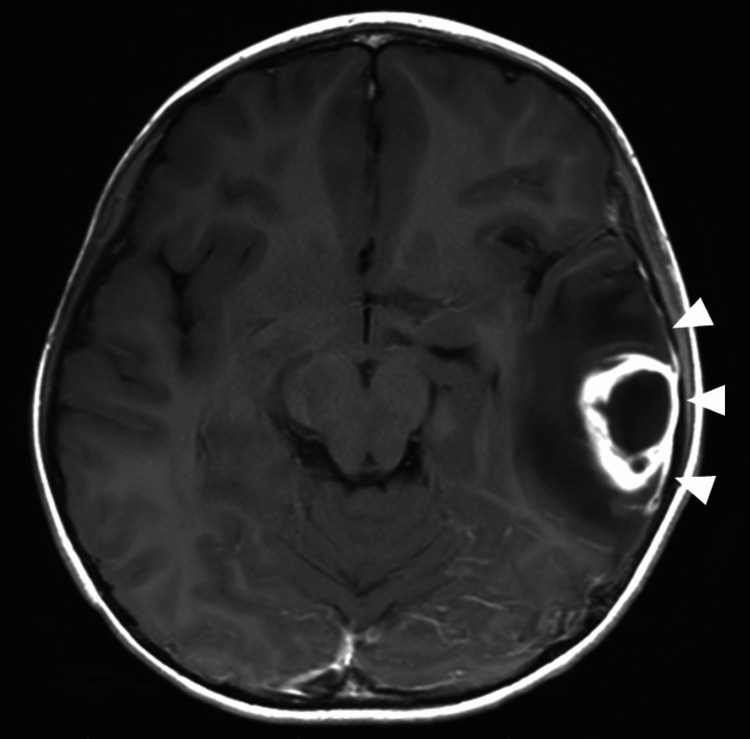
Magnetic resonance image of patient's brain. Axial T1-weighted magnetic resonance image with intravenous contrast showing a ring-enhancing abscess (32 mm × 24 mm) in the left temporal lobe associated with inflammation in the adjacent dura mater (white arrowheads).
